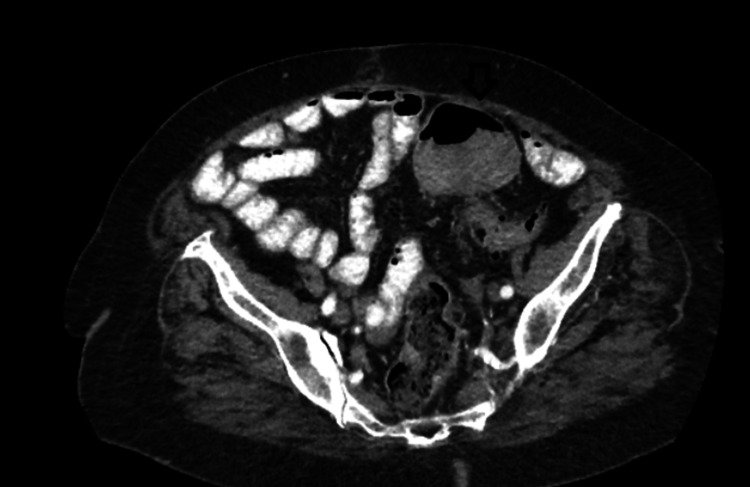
CT scan revealing distended loops and a mass almost obstructing the lumen
